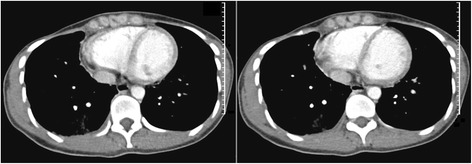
HRCT scan of the patient before thoracoscopy. Small amount of unilateral pleural effusion was detected on the right side of the patient. None visible pleural nodes were reported. (Opacity showed in the patient’s right lung which has been proved to be bacterial infection because it disappeared after treatment of antibiotics)
